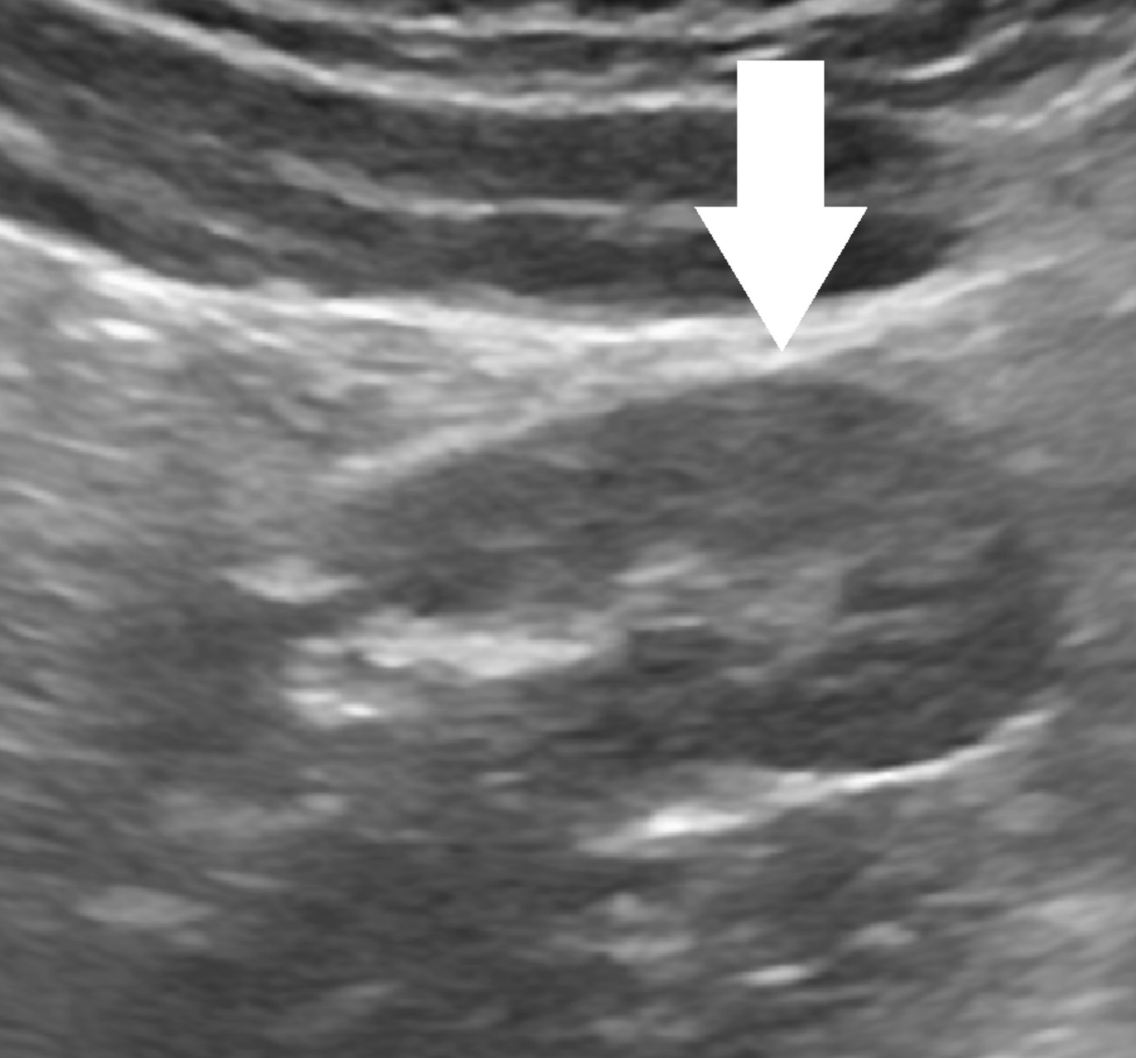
Grayscale Ultrasound Image Grayscale ultrasound image demonstrating a centrally hyperechoic and peripherally hypoechoic right lower quadrant mass without signs of inflammation.
